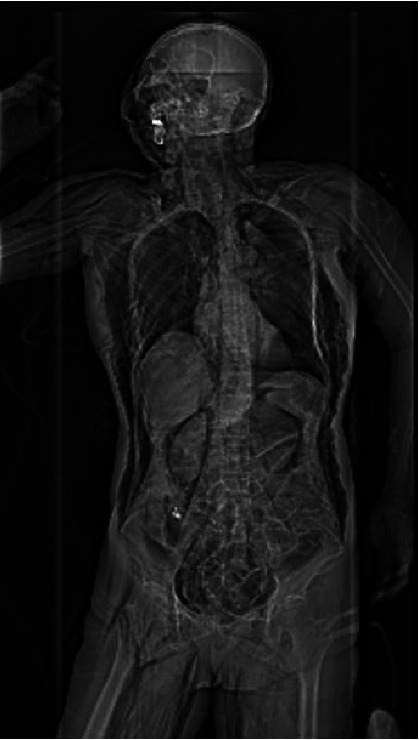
CT scanogram showing subcutaneous emphysema in the face, neck, chest, abdomen, and upper thighs.
